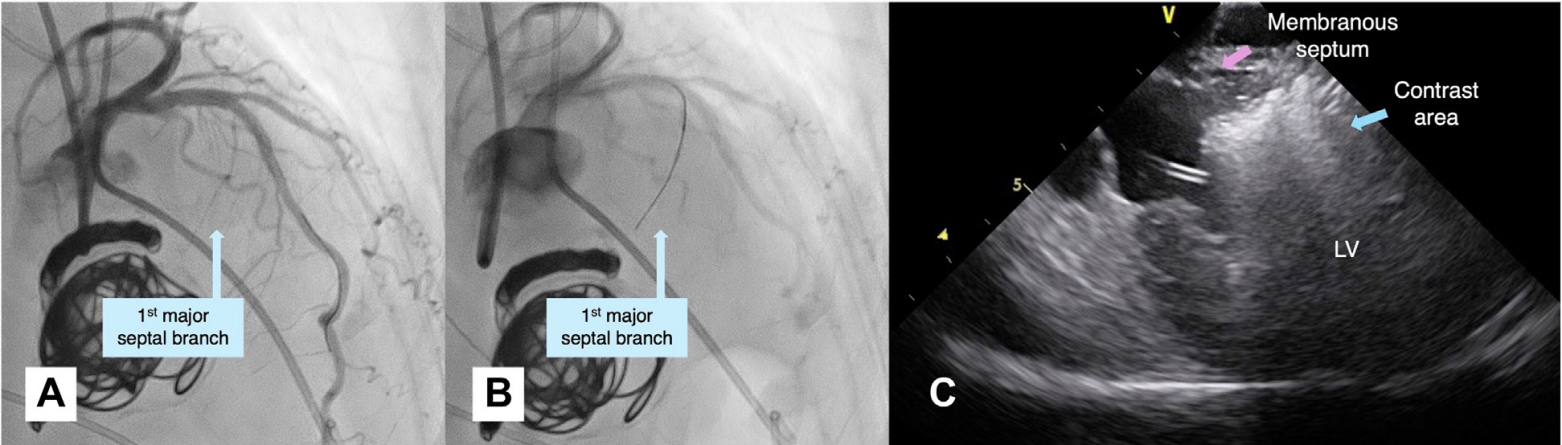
Imaging in Case 4 (A and B) Coronary angiography (A) before ablation and (B) during wiring to the first branch from the left anterior descending artery. (C) Jugular vein intracardiac echocardiography showing an ethanol injection to the first branch from the left anterior descending artery. No high signal intensity is observed in the membranous septal region. LV = left ventricle.
